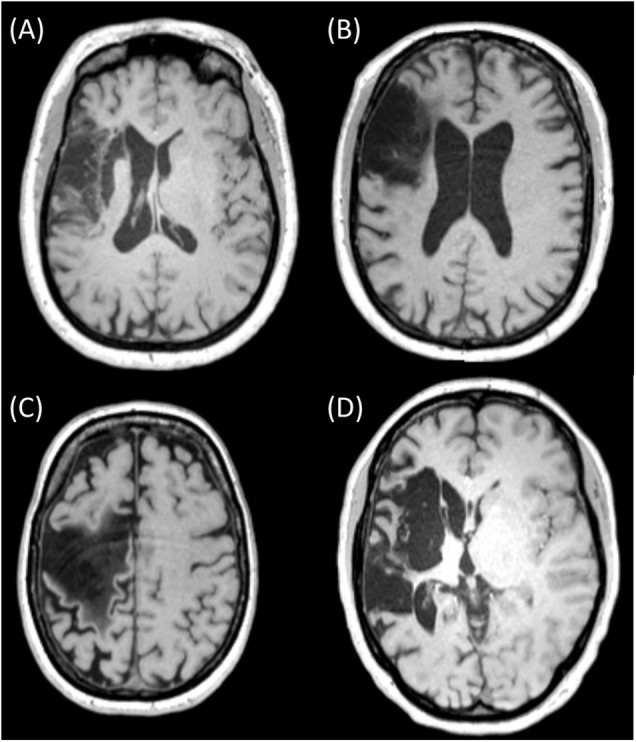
MRI slices of four different chronic stroke subjects showing lesions of various sizes and mixed composition [(A) (subject 042), (B) (subject 034), and (C) (subject 051)] and with primarily CSF [(D) (subject 055)]. Ethical approval was acquired to record and publish the MRI slices with consent from the participants (see NL58437.091.17).
